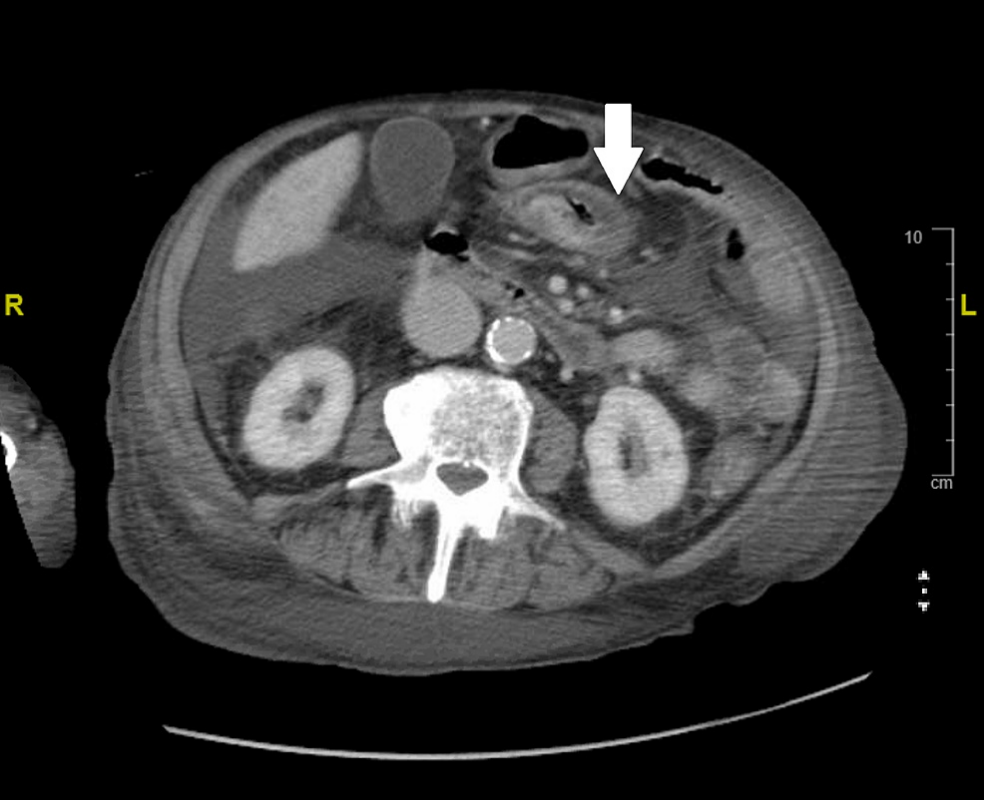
Computed tomography scan of the abdomen showing bowel wall thickening and surrounding inflammatory changes consistent with colitis.
